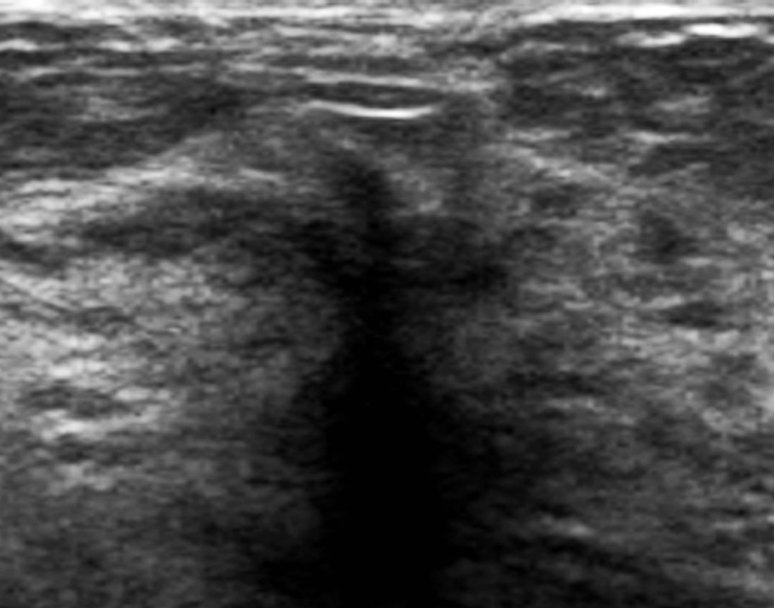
Ultrasound. Ultrasound demonstrates a vague area of focal shadowing and distortion without a discrete mass. Pathology showed invasive lobular carcinoma.
